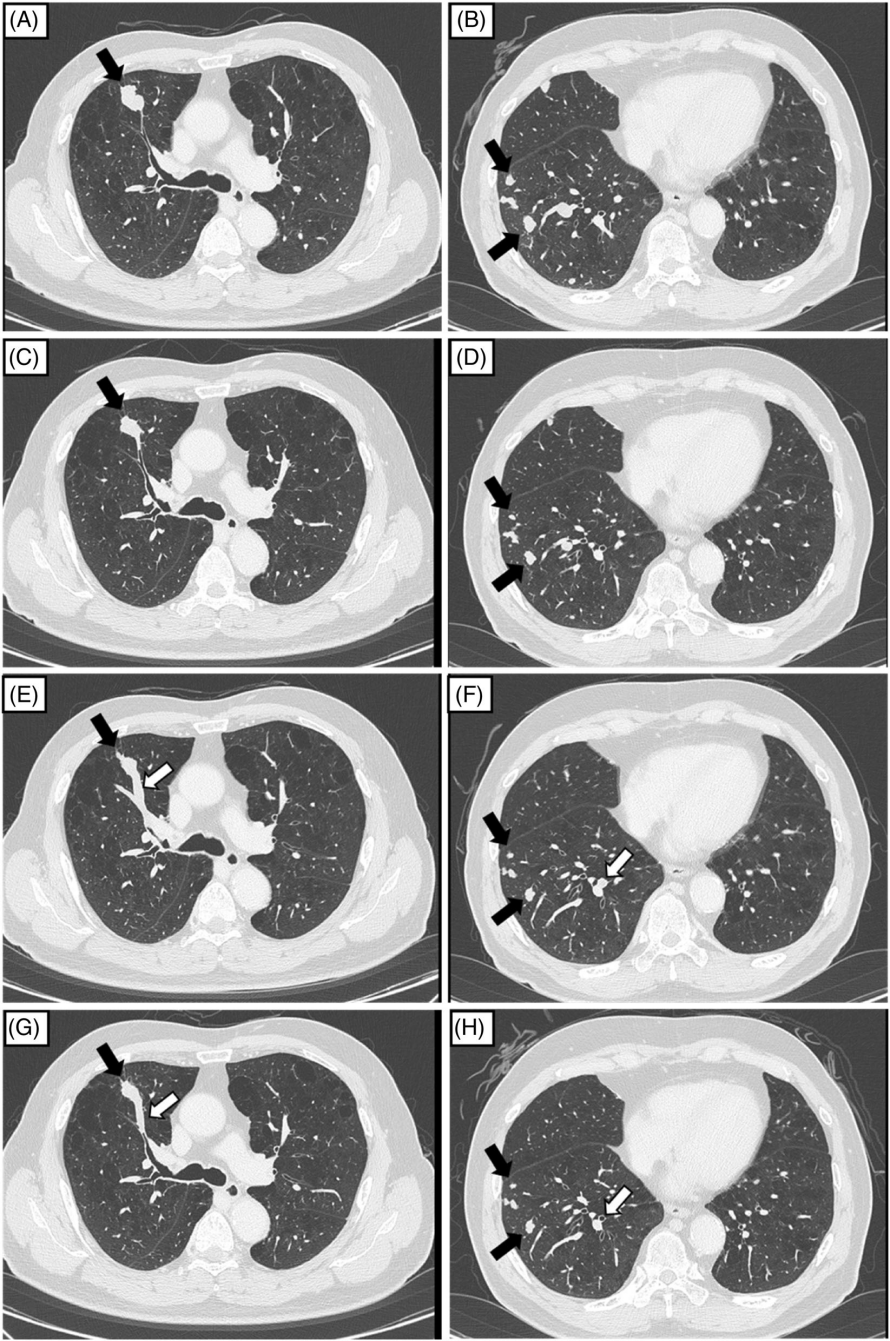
Chest computed tomography (CT) revealed that bilateral multiple lung metastases (black arrows) were found in all lung fields, 2 years after liver lobectomy (A, B). After four cycles of combination therapy with atezolizumab and bevacizumab, multiple lung metastases were reduced (C, D). After 23 cycles of the combination therapy, chest CT showed that soft tissue shadows (white arrow) appeared within the right upper and lower bronchi. However, lung metastases were maintained without an increase in the size of the existing lesions or the appearance of new lesions (E, F). After lenvatinib induction, endobronchial metastases were reduced (G, H).
